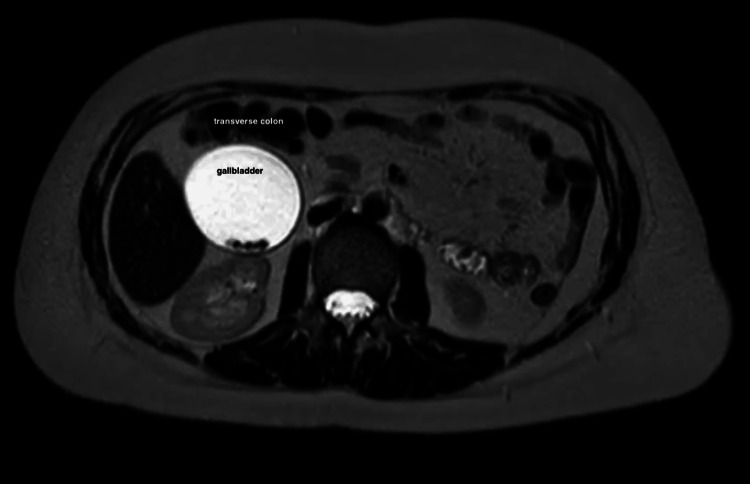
MRI showing the infracolonic location of the gallbladder
